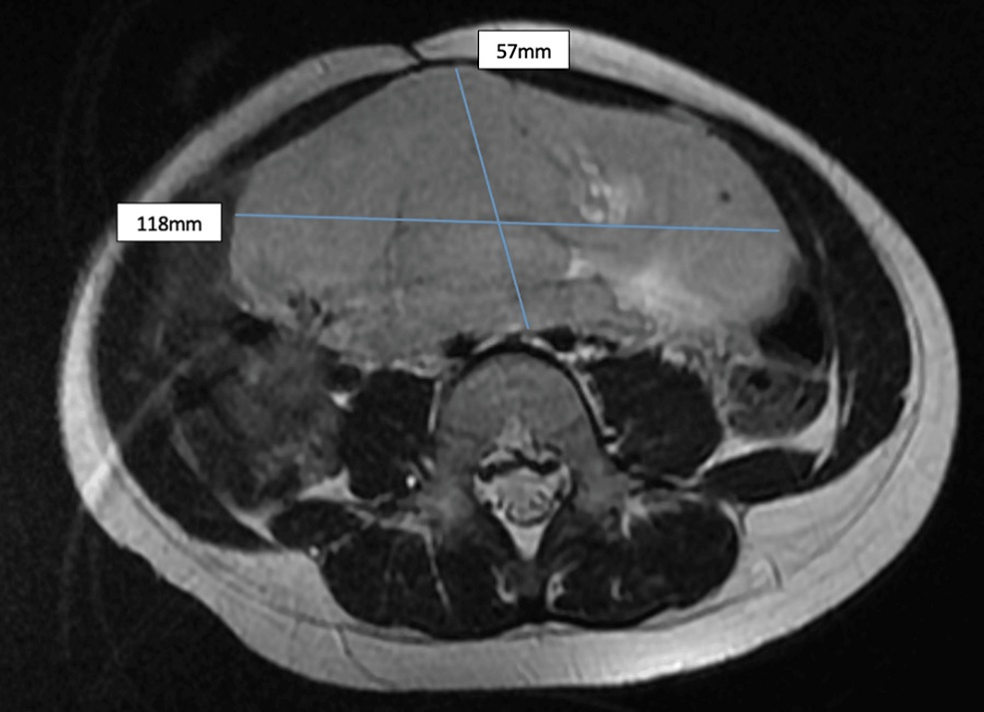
Pelvic MRI showed the presence of a voluminous right ovarian mass, lobulated in outline. The blue lines show the limits and dimensions of the tumors.
